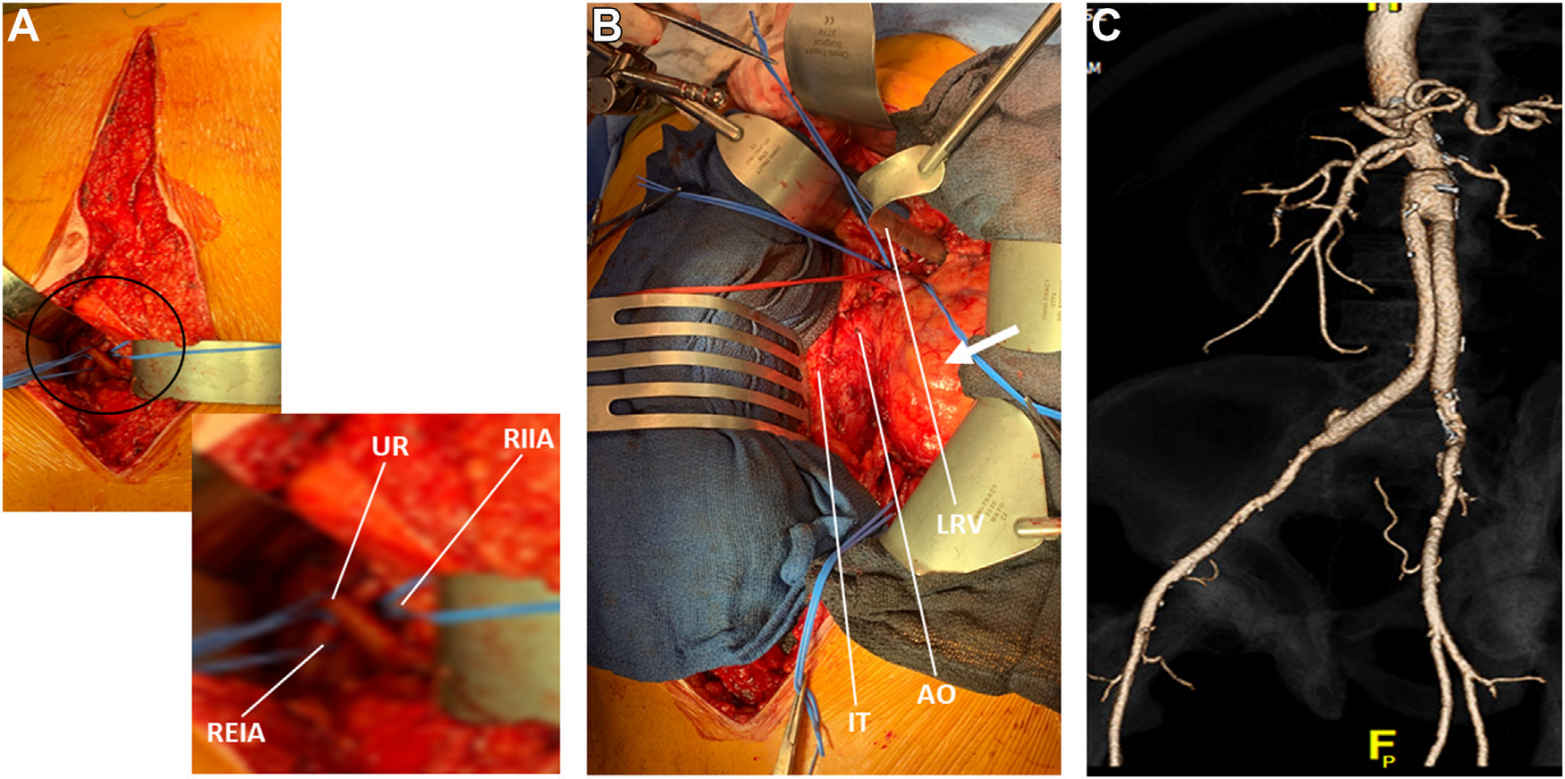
A, Surgical control of the right common iliac artery bifurcation. The right anterior rectus sheath was divided 3 cm from the midline, the rectus muscle was retracted laterally, and the pre- and retroperitoneal planes were developed bluntly until the iliac bifurcation had been identified medial to the psoas muscle. B, The paravisceral aorta was exposed and all mesorenal branches were controlled with vessel loops. C, Three-dimensional computed tomography scan at 1 year postoperatively showing a widely patent graft, visceral branches, and anastomoses. *REIA,* right external iliac artery; *RIIA,* right internal iliac artery; *UR,* ureter.
